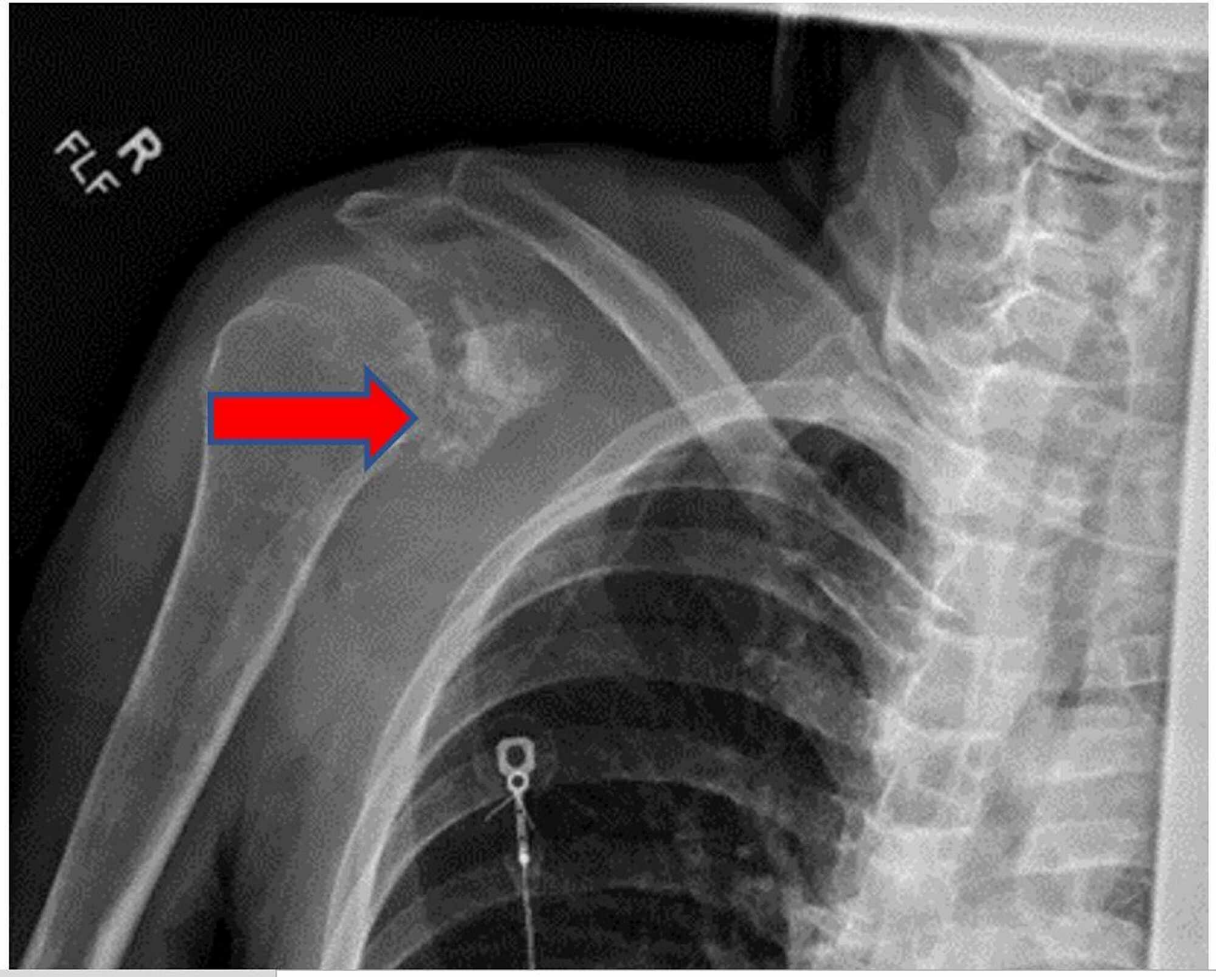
Right shoulder plain x-ray revealing bony destruction of scapula, (red arrow).
